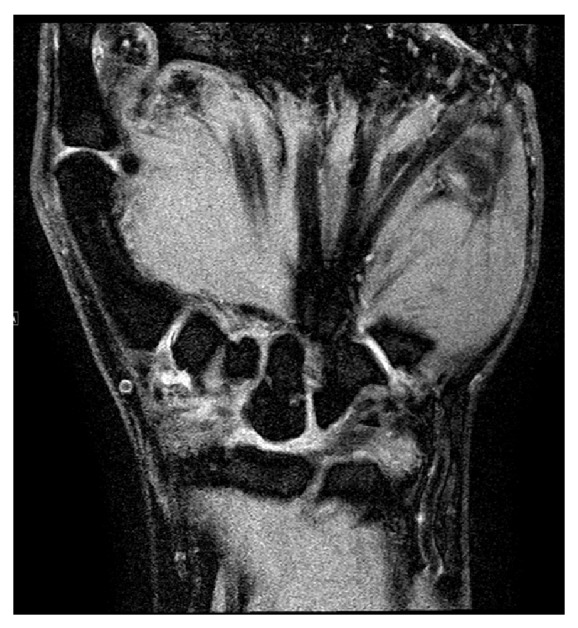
Post-op MRI: no significant chondropathy in fossa lunata or at proximal capitate and no bone marrow edema.
